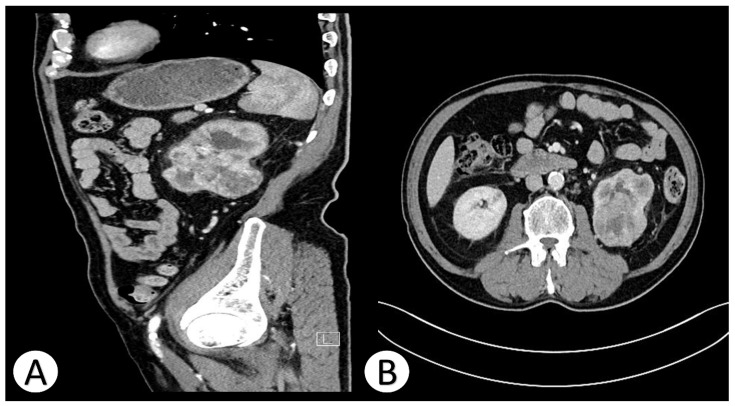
Contrast-enhanced CT of the patient’s abdomen, shown in sagittal (A) and axial (B) views (the white letter “L” denotes the left side).
